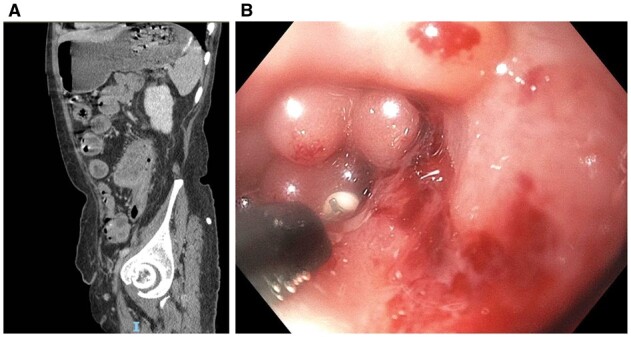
Removal of lumen-blocking inflammatory polyps. (A) Large intraluminal polyps in the descending colon on computed tomography; (B) endoscopic polypectomy.
